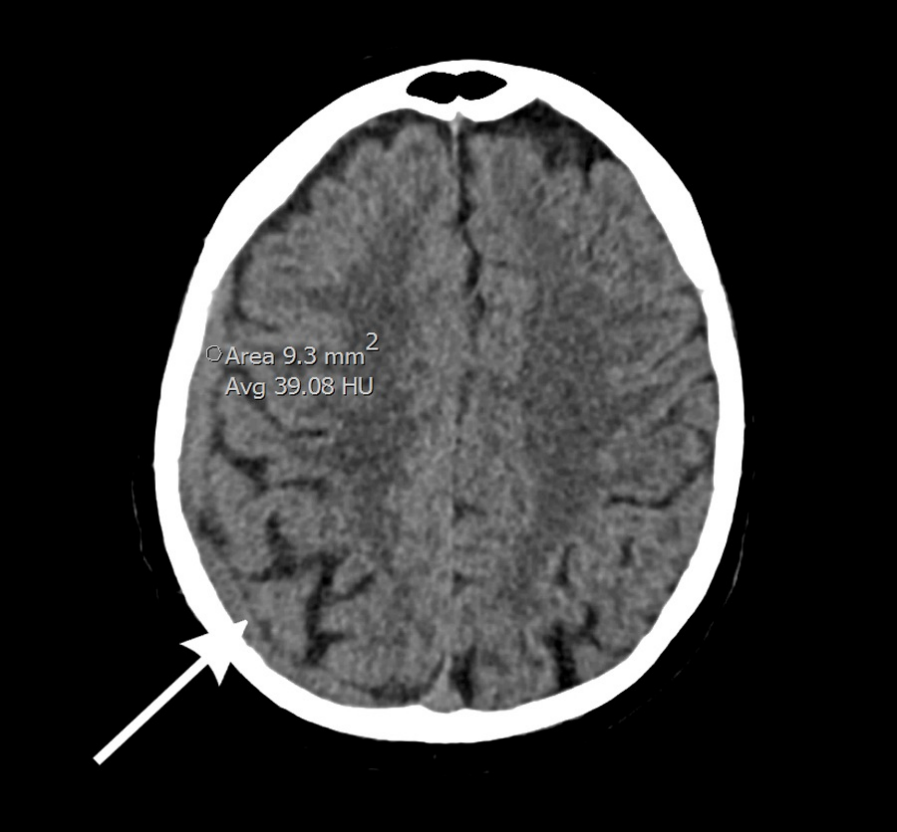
Computed tomography (CT) of the head shows a diffuse, enhancing dural thickening overlying the right hemisphere with focal areas of nodularity, suggestive of metastatic disease Associated mass effect is seen with 6 mm of left-sided midline shift.
